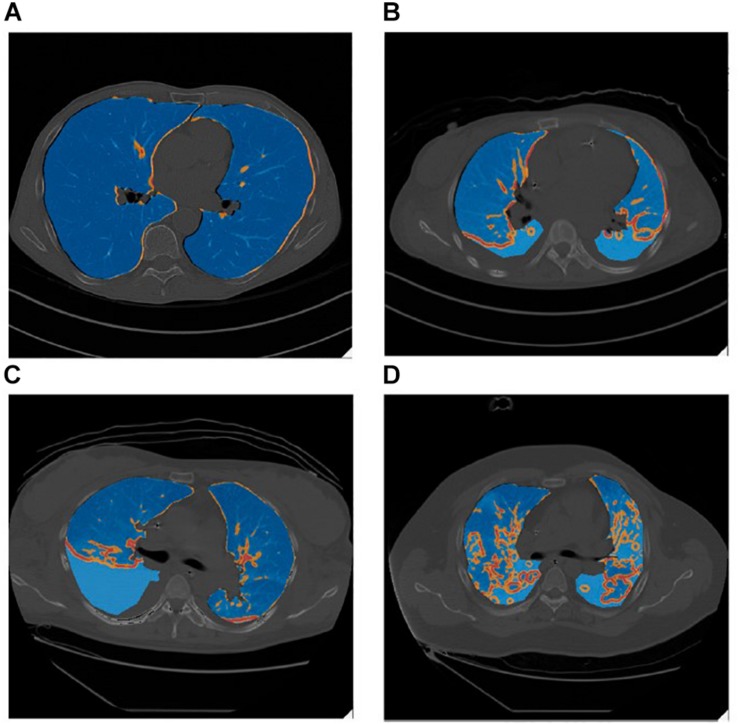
Lung inhomogeneity can be visualized grossly on CT scan as evident in these false-color images in which stress-raisers are color mapped (blue = regions of mild stress-raisers, orange = regions of moderate stress-raisers, red = regions of severe stress-raisers). Blue intensity differences are due to overlapping between the underlying CT scanning images and the false-color map of lung inhomogeneities. (A) Represents a control subject with healthy lungs taken at end-inspiration. (B) a patient with mild ARDS, (C) a patient with moderate ARDS, (D) a patient with severe ARDS, all taken during the expiratory phase with a PEEP of 5 cmH_2_O. The regions of stress-risers expose the neighboring alveoli to nearly double the applied transpulmonary pressure (Cressoni et al., 2014). *Published with permission.*
